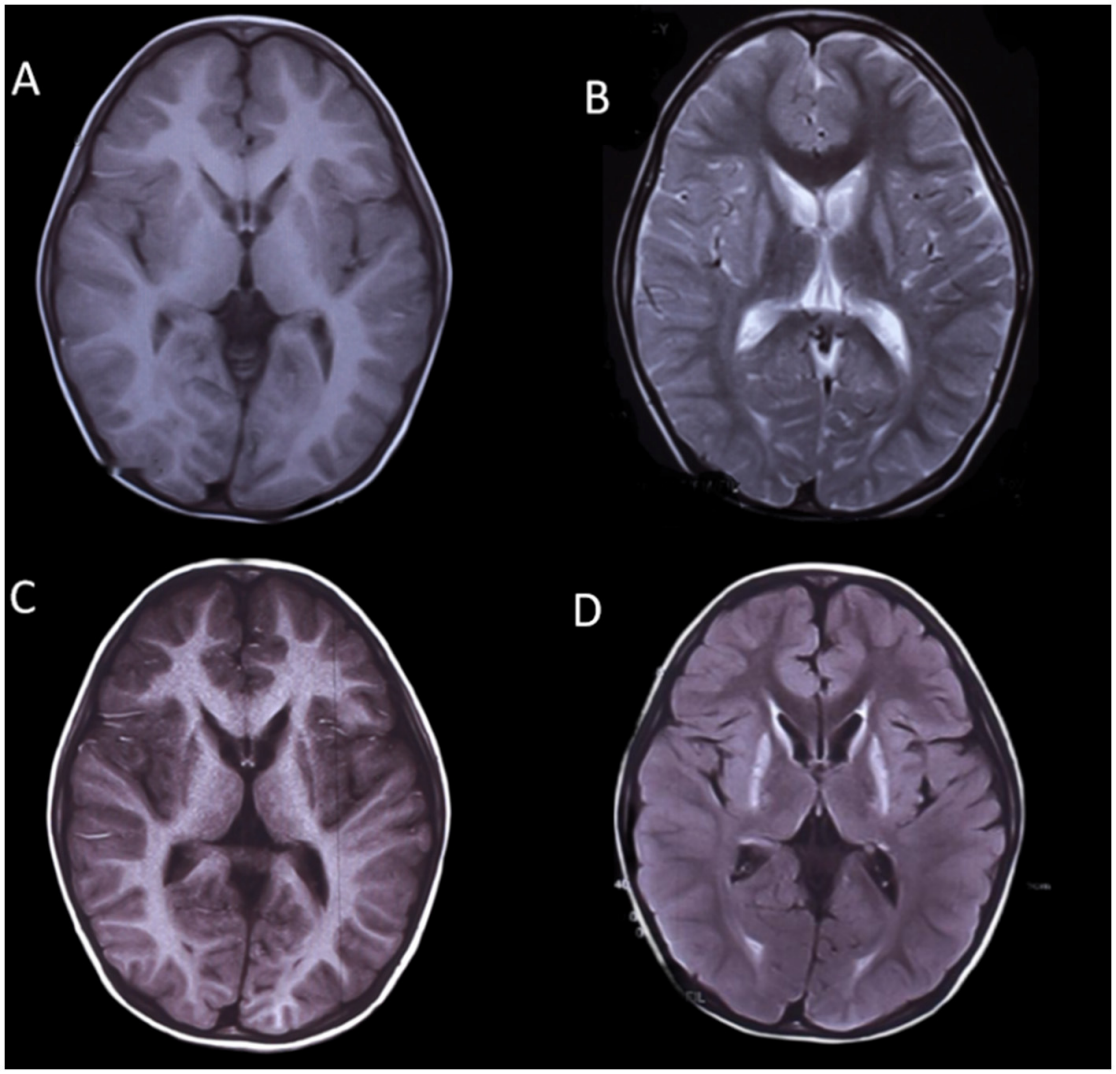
MRI images of a boy with POLR3-HLD (Patient 2), (A, B) at 4 years of age and (C, D) at 7 years of age. The MRI image shows T1-hyperintense and T2-hyperintense of bilateral caudate nucleus and lentiform nucleus.
